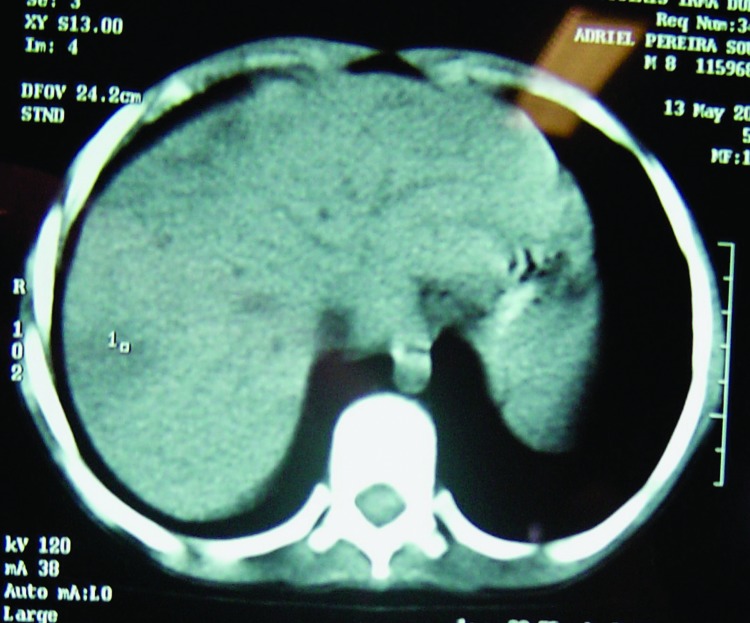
Computed tomographic scan of abdomen of patient 3, showing multiple, small liver abscesses.
